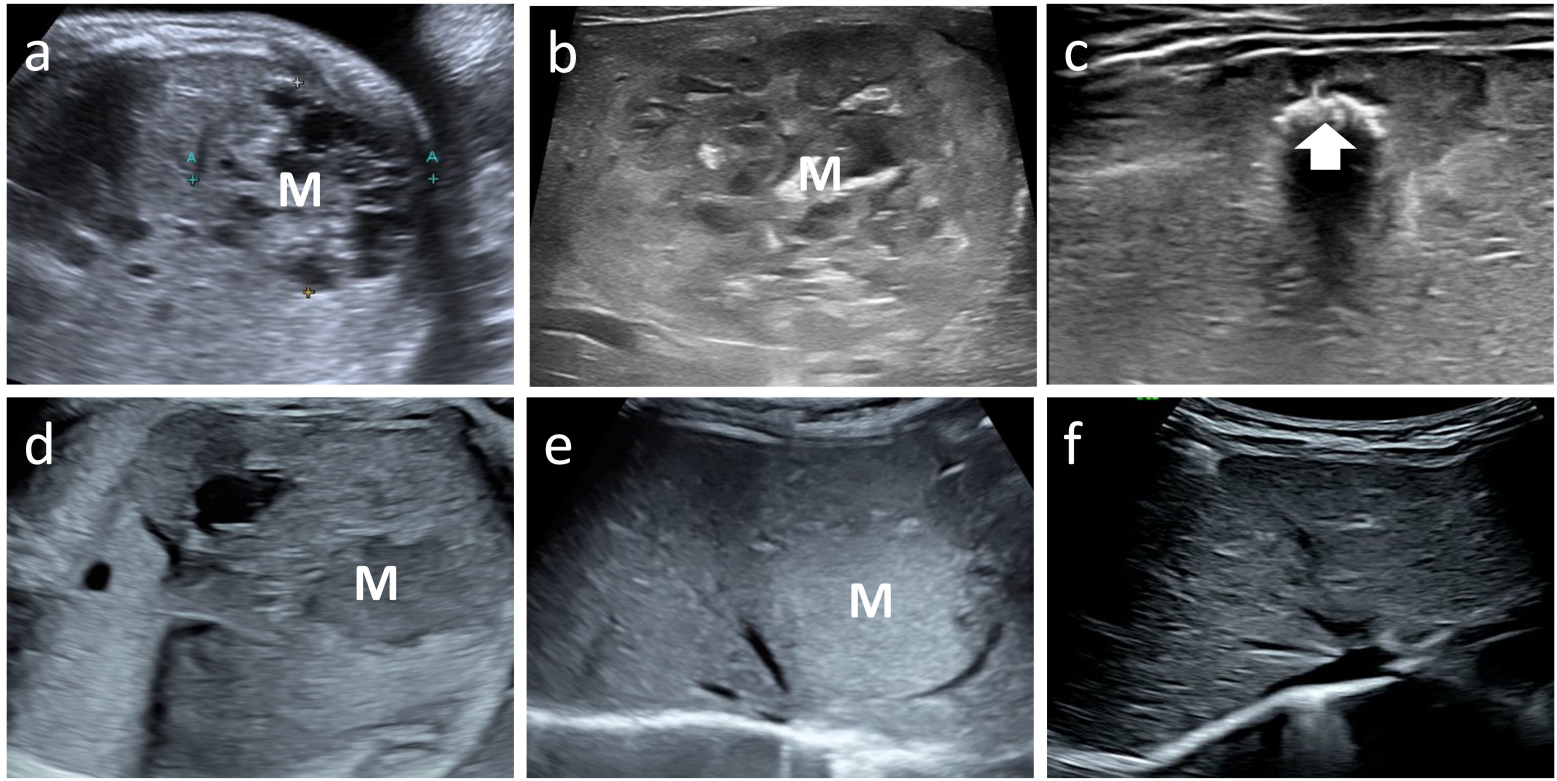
Case 6 and 8 with mass (M) on fetal ultrasound, postnatal ultrasound with maximum volume and follow up image showing regression. Case 6 fetal and postnatal images (a, b) show internal heterogeneous, honeycomb hypoechogenicity. The tumor volume peaked at 112 ml. The final regression image (c) shows patches of echogenic calcification (arrow). Case 8 fetal and postnatal images (d, e) show a homogeneous mass, hypoechoic on prenatal ultrasound and hyperechoic on postnatal ultrasound. The tumor volume peaked at 13 ml. The tumor eventually regressed completely (f).
